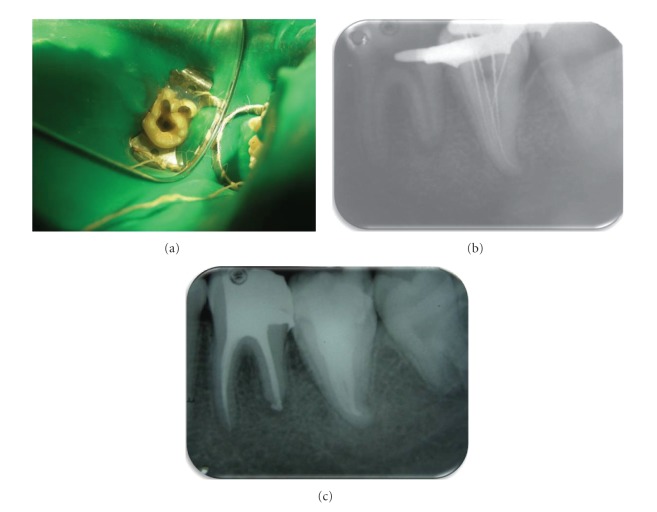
(a) Occlusal view. (b) Working length determination. (c) Postoperative final radiograph.
